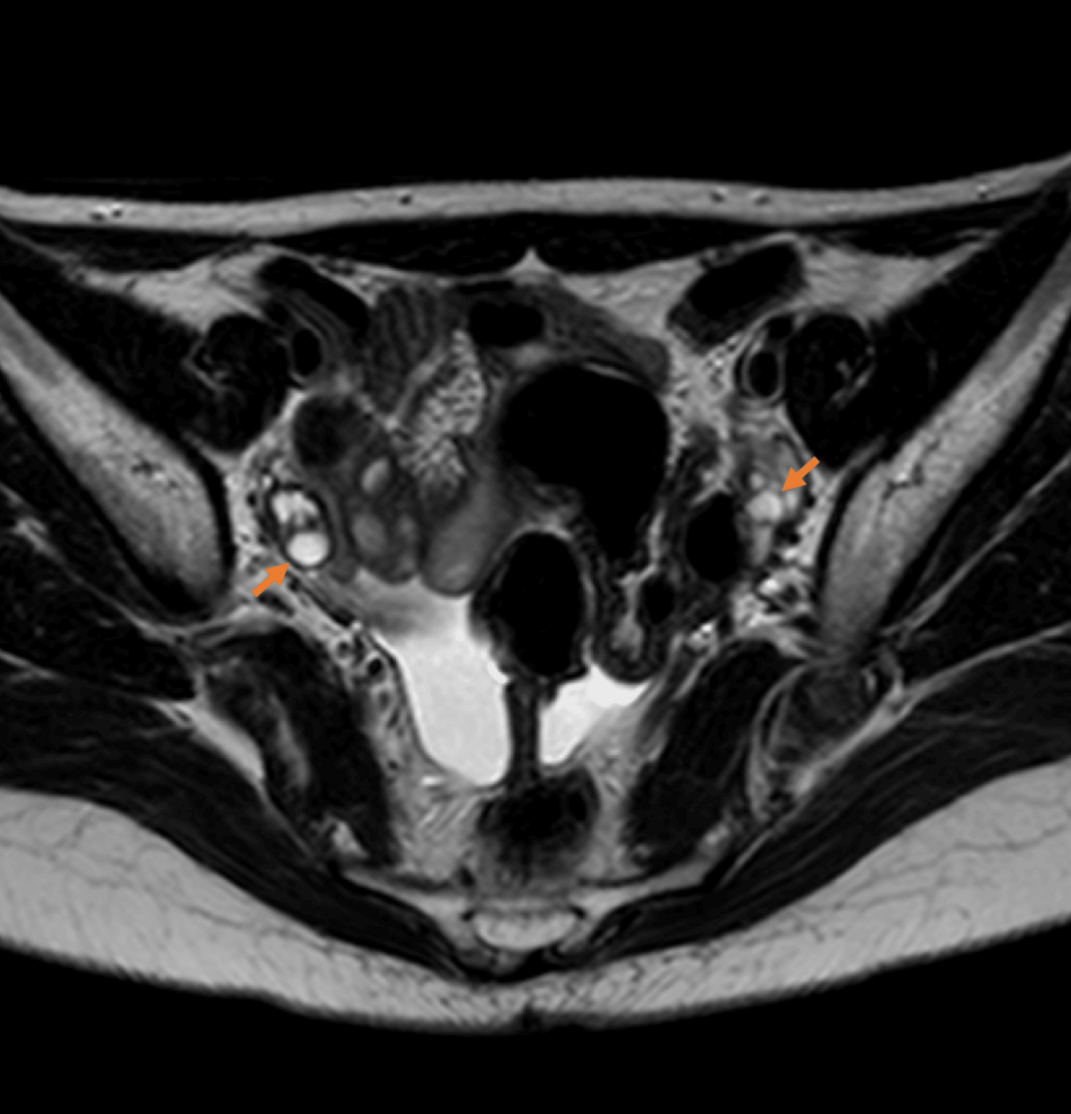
Magnetic resonance imaging (MRI) radiographs showing small follicles (orange arrows)
